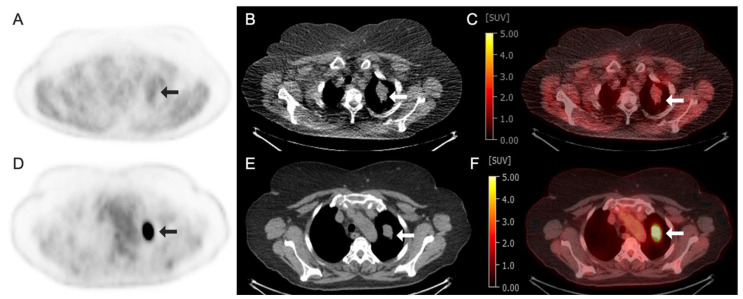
Images obtained from a 70-year-old woman with non-small-cell lung cancer demonstrating radiotracer-avid primary lung neoplasm at the left lung apex (arrows). (A–C) Fluorine 18 (^18^F)-labeled Fluoromisonidazole (FMISO) PET/CT images. (A) ^18^F–FMISO axial PET image; (B) axial CT image; (C) axial fused ^18^F-FMISO PET/CT image with an SUV max of 2.5. (D–F) Fluorine 18 (^18^F)-labeled Fluorodeoxyglucose (FDG) PET/CT images of the same patient performed 1 week prior. (D) ^18^F-FDG axial PET image; (E) axial CT image; (F) axial fused ^18^F-FDG PET/CT image demonstrating intense avidity with an SUV max of 24.
